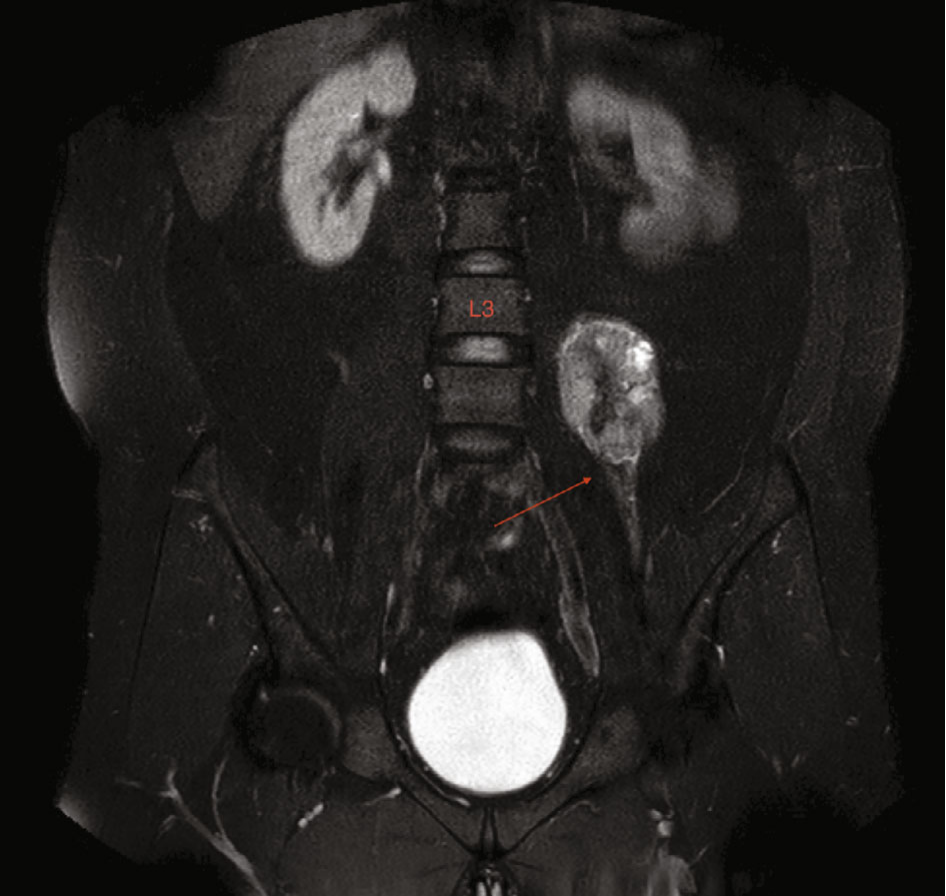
The red arrow showing the course of the femoral nerve intersecting the inferior border of the tumor. L3: third lumbar vertebra (coronal T2-weighted MRI).
